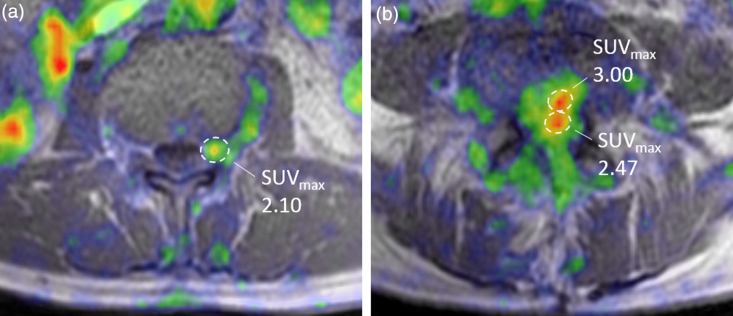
Example case of a patient with LBP, showing increased [^18^F]FDG uptake in two locations. (a) Increased uptake in the left lateral recess at level L2. (b) Abnormally increased uptake in a herniated intervertebral disc at level L4–L5, as well as the spinal canal directly posterior to the disc. [^18^F]FDG, [^18^F]fluorodeoxyglucose; LBP, low back pain; SUV_max_, maximum standardized uptake value.
